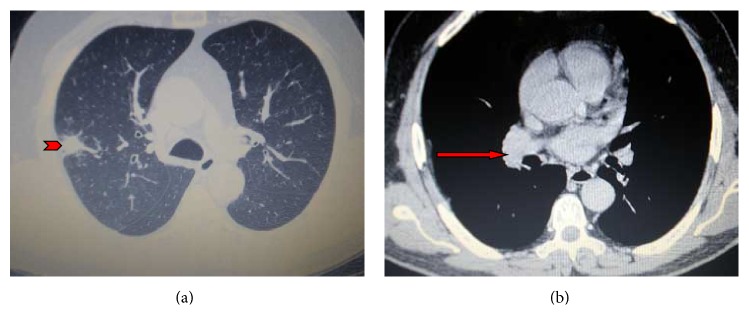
(a and b) Contrast-enhanced chest computed tomography showing a nodular opacity in the posterior segment of the right upper lobe (arrowhead) accompanied by mild ipsilateral pleural thickening and bilateral mediastinal lymphadenopathy (red arrow).
